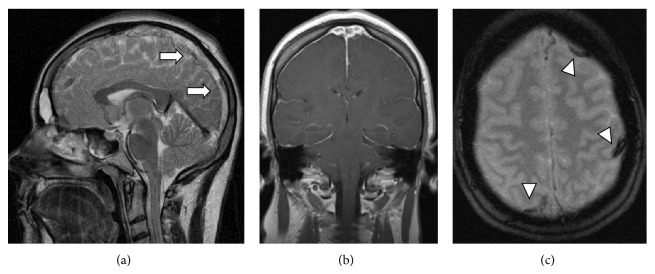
Brain MRI. (a) Sagittal T2-weighted image, (b) coronal T1-weighted image, and (c) axial gradient-echo image show superior sagittal sinus thrombosis (arrow) and thrombosis of multiple cortical cerebral veins (arrowheads).
